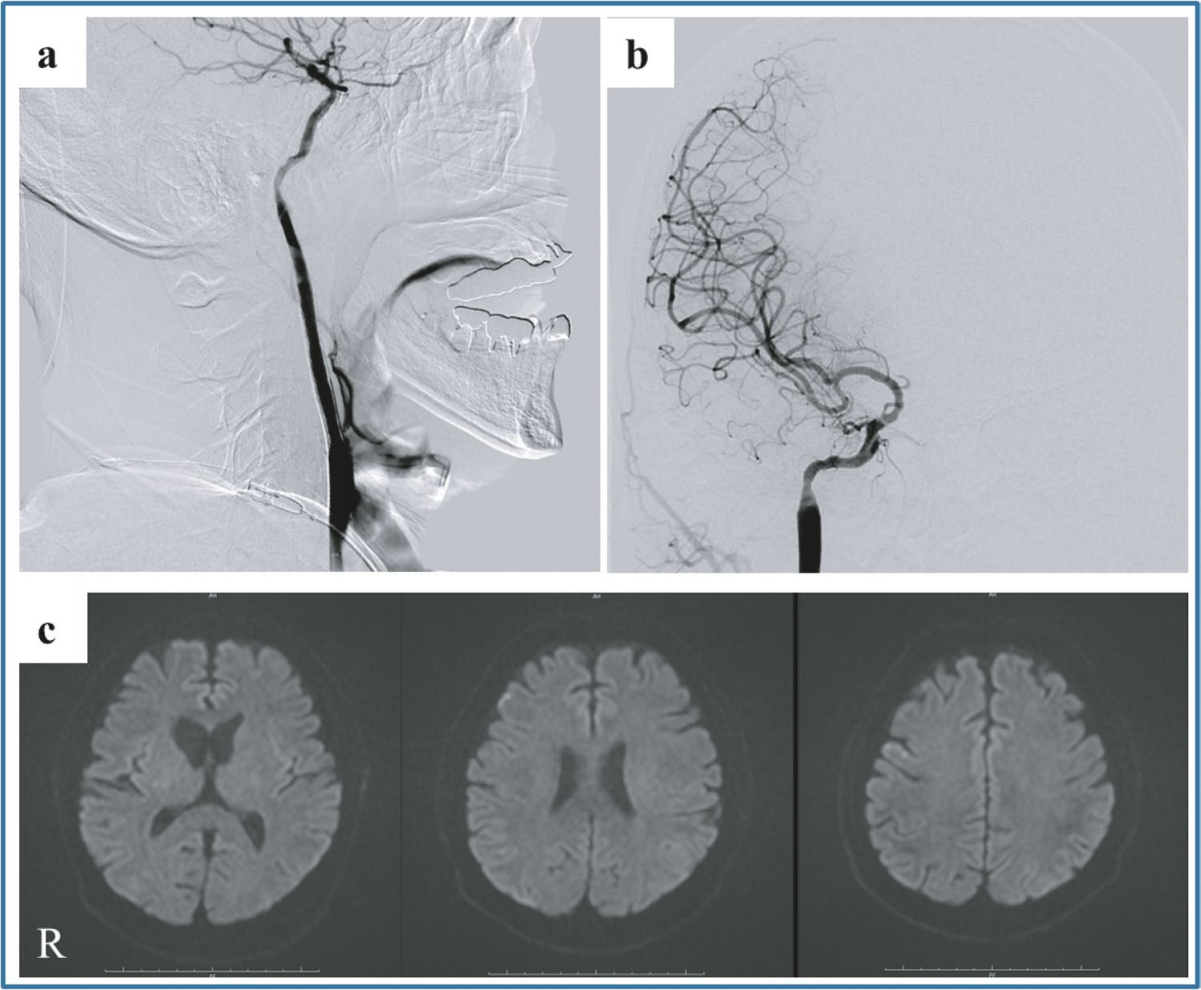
Image after treatment. (a–b) The right common carotid angiography revealed patency of the right internal carotid artery after 3 months. (c) Diffusion-weighted magnetic resonance image 1 day after the procedure revealed small ischemic lesions in the ipsilateral cerebral hemisphere
